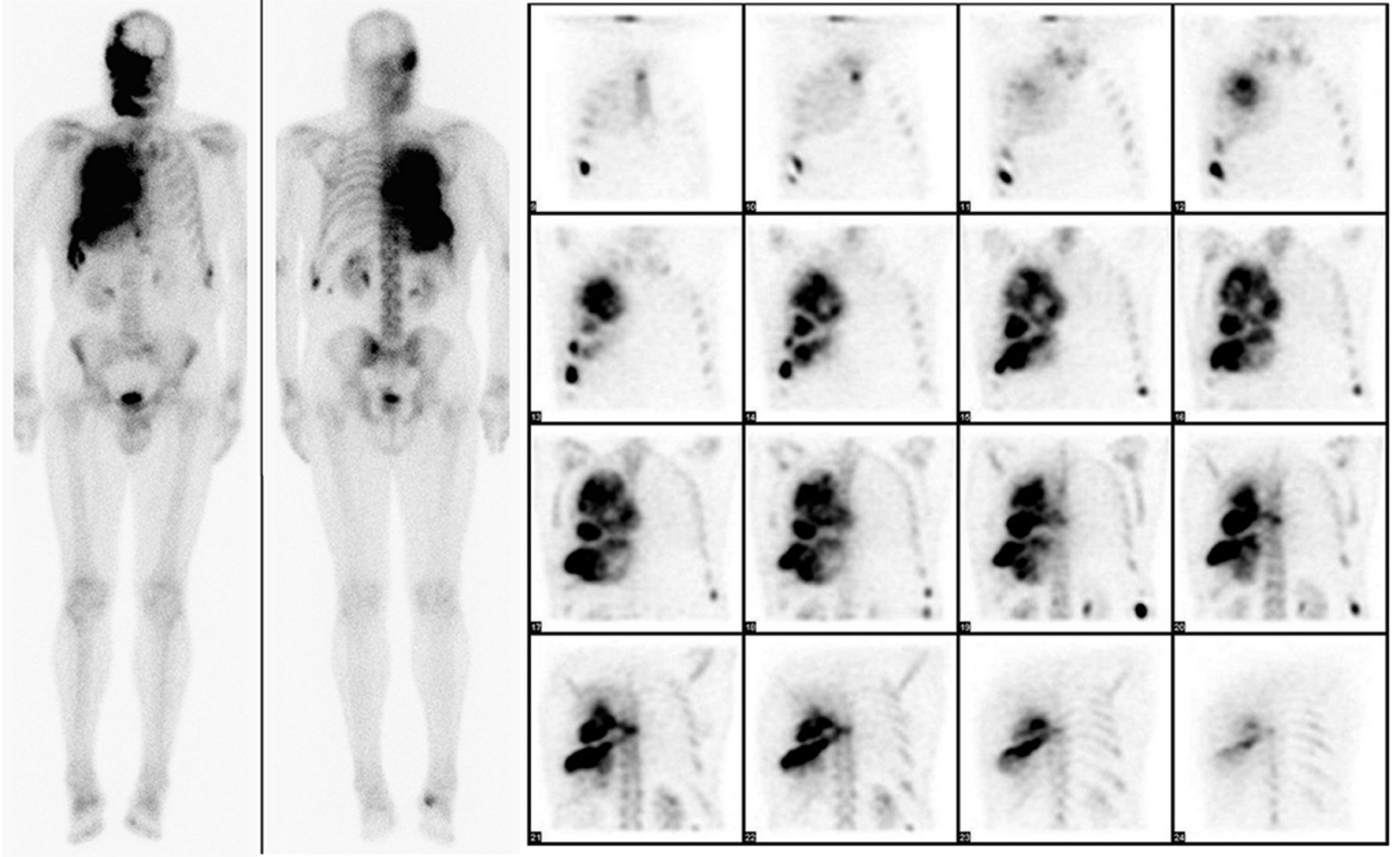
^99m^Tc-methylene diphosphonate whole-body bone scan showing intense uptake in several right facial bones, including the frontal, zygomatic and nasal bones, maxilla and mandible. There was also intense uptake involving the right ribcage. Whole-body planar anterior and posterior images and single-photon emission CT coronal images of the thorax are shown.
